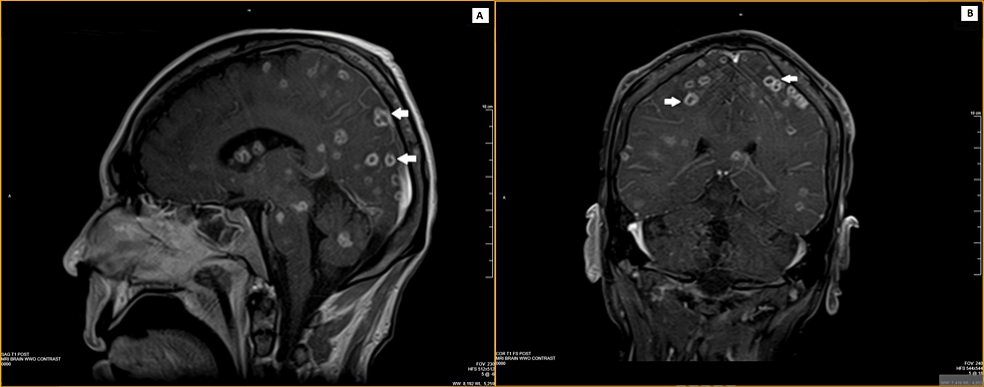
MRI brain before biopsy (T1-weighted) showing multiple ring-enhancing lesions (white arrows) on the (A) sagittal section and (B) coronal section. MRI, magnetic resonance imaging
